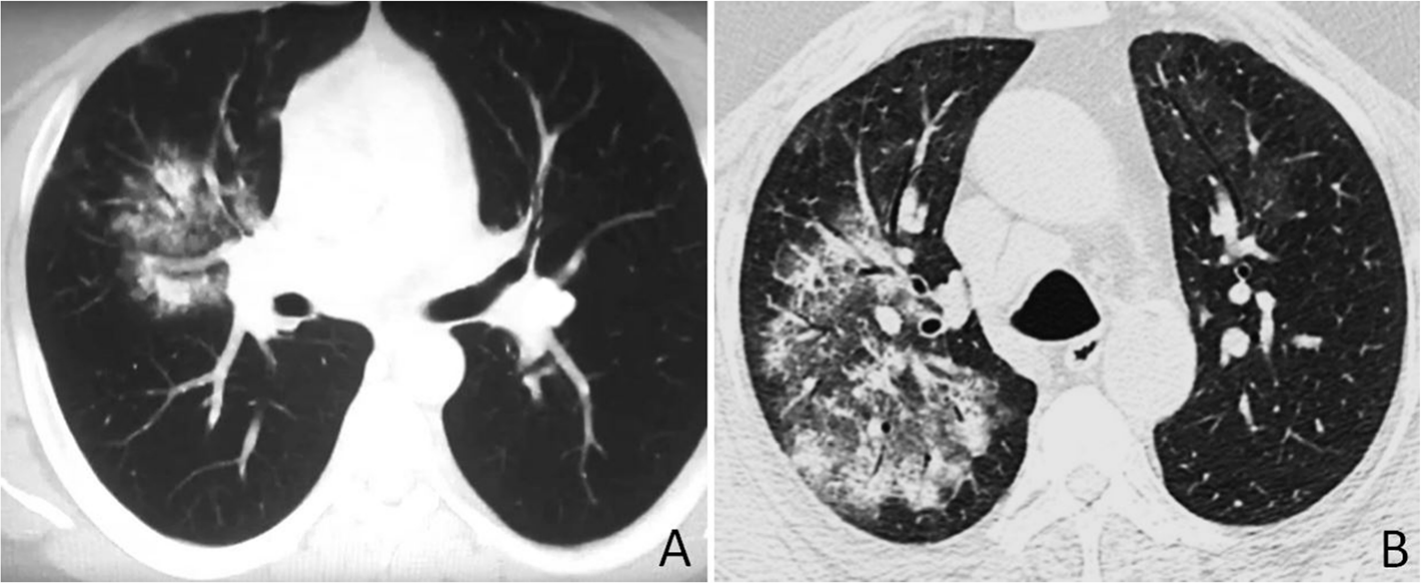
COVID-19 and cryptogenic pneumonia misdiagnosed as COVID-19. a Axial CT image obtained from a 33-year-old man with positive PCR test for COVID-19 shows area of clearing consolidation with central ground-glass density “reversed halo sign” in the right lung. b Cryptogenic organizing pneumonia in a 55-year-old man with history of chest infection not responding to multiple courses of antibiotics; axial CT image shows reversed halo sign in the right lung with small areas of GGO in the left lung. Transbronchial biopsy showed findings of COP
